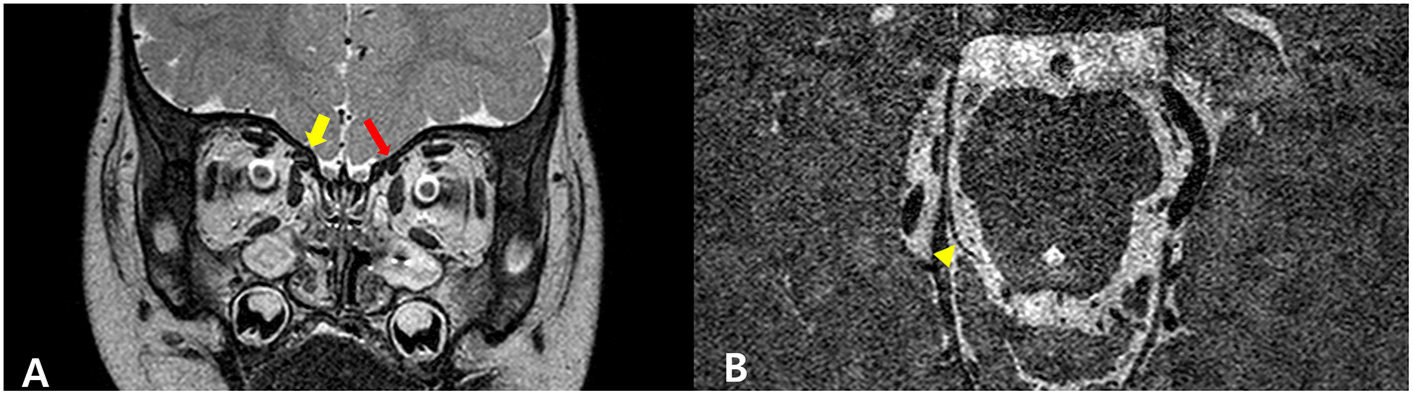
Hypoplasia of the left superior oblique muscle and an absence of the left fourth cranial nerve. Coronal T2-weighted orbital image showing that the left superior oblique muscle (red arrow) is smaller than the right (yellow arrow), suggesting hypoplasia (A). A high-resolution image shows that the right fourth cranial nerve (arrow) is well-identified; however, the left fourth cranial nerve is not visible (B).
